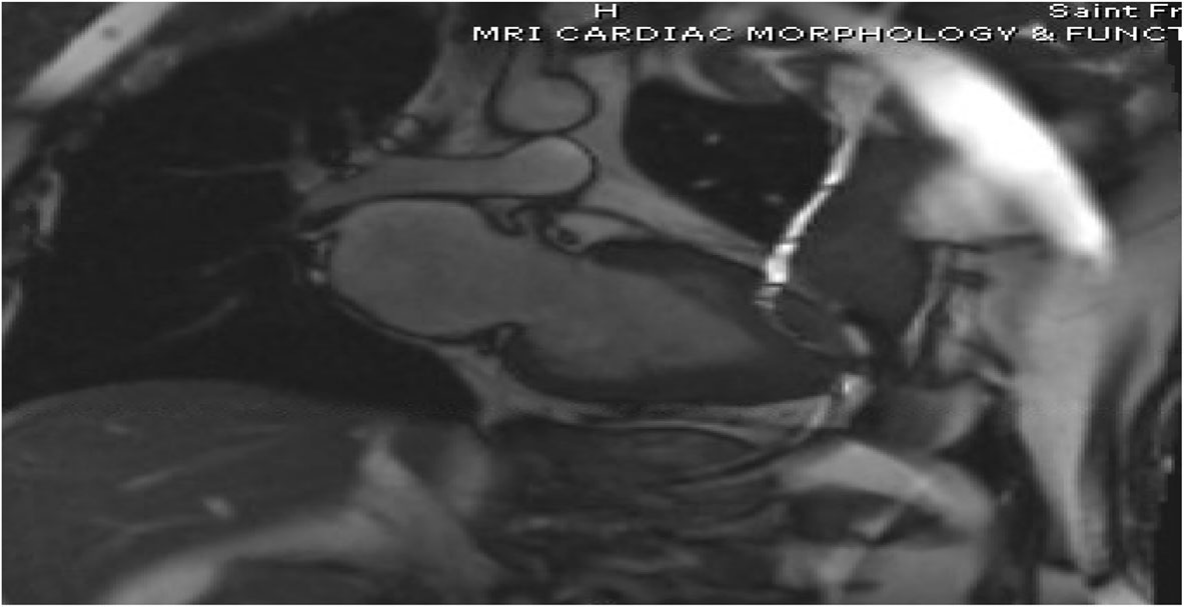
MRI imaging revealing severely thickened apical myocardium with “spade” appearance
